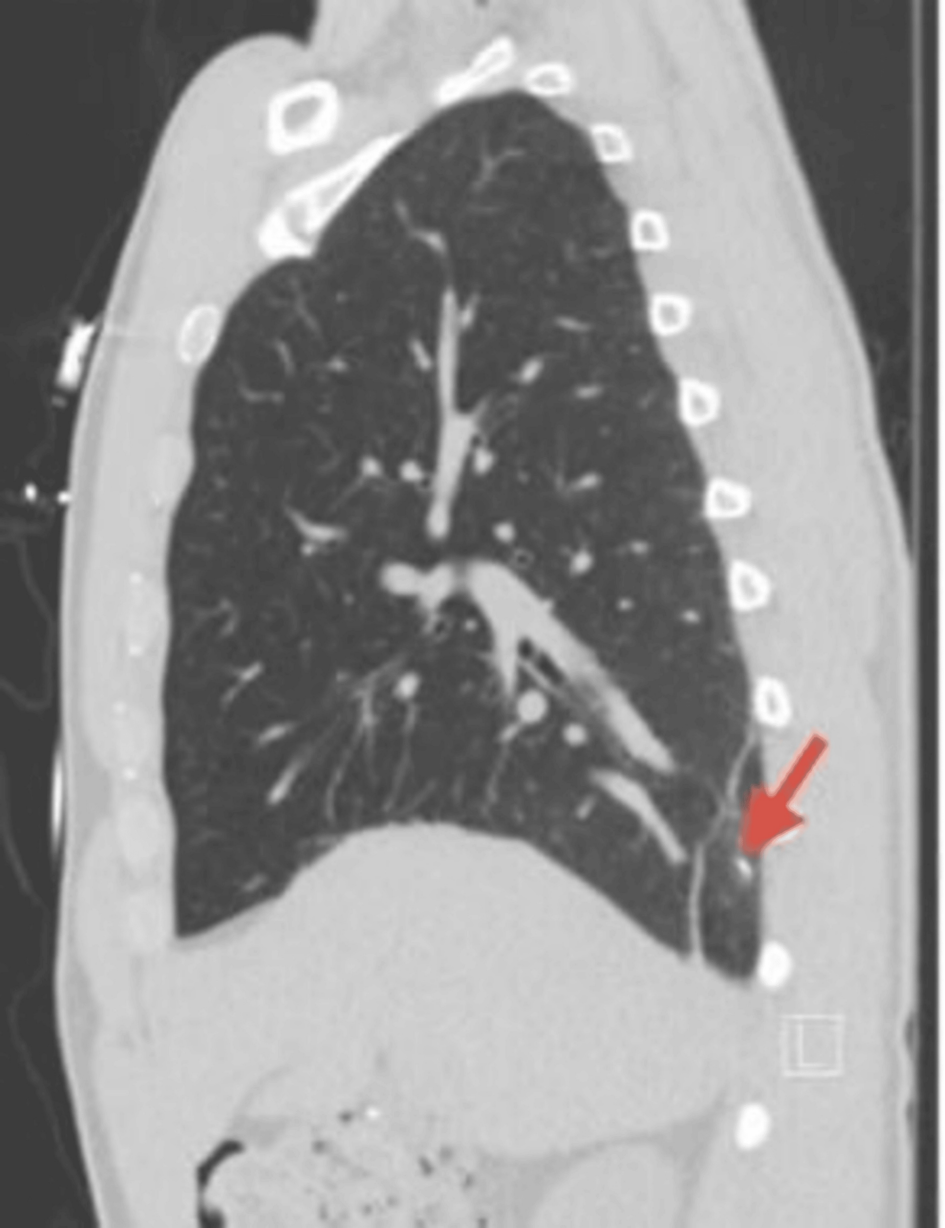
CT scan with an arrow pointing to the retained injection needle (sagittal view)
